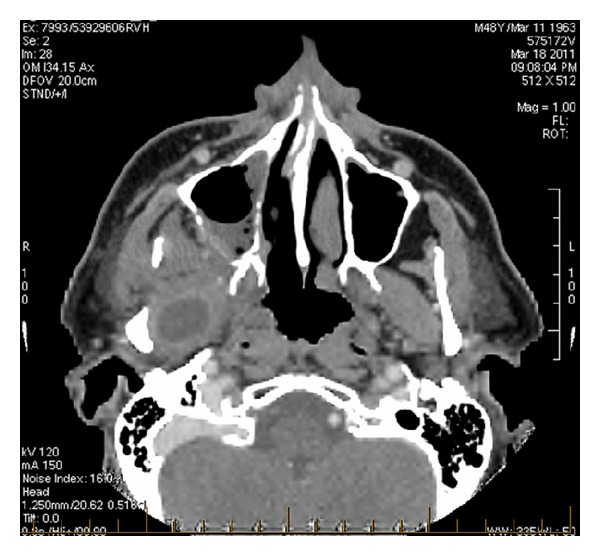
CT axial cut of patient A. V. Right-sided erosion of the posterior wall of the maxillary sinus and a 3.0 cm right lateral pterygoid muscle abscess in the masticator space of the ITF.
